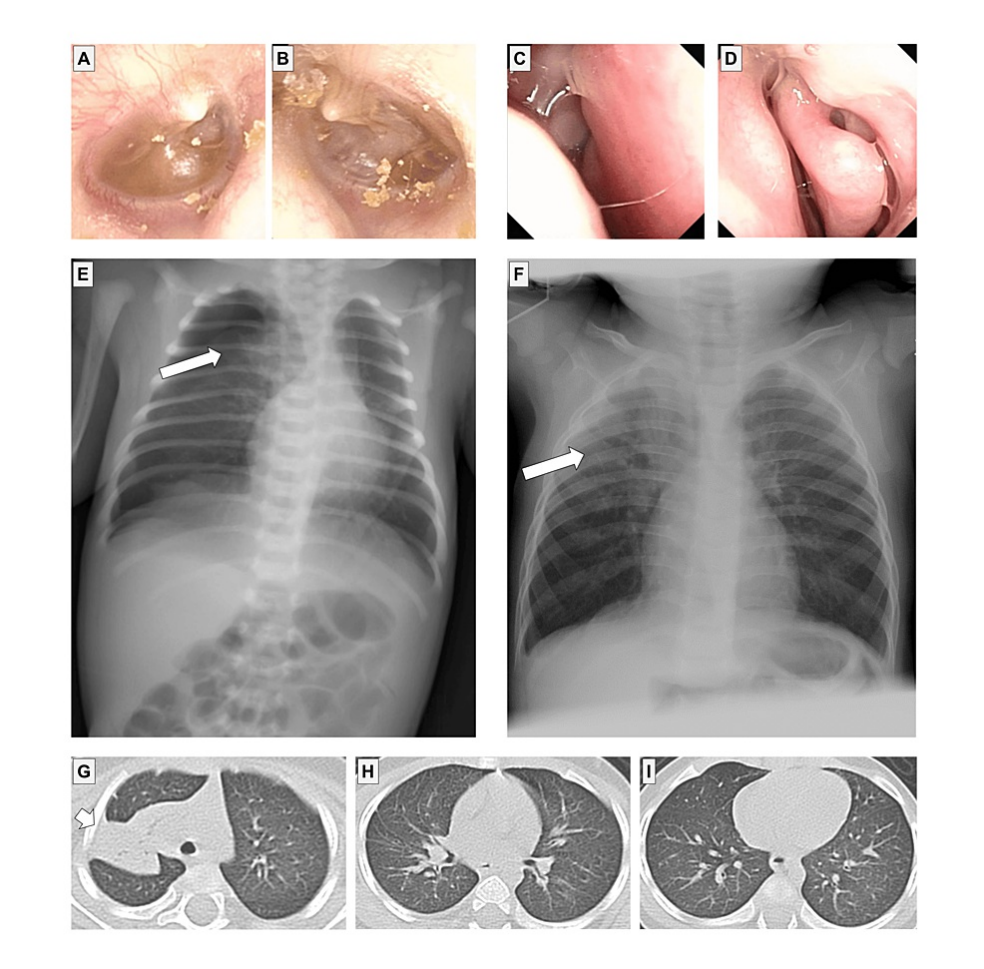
Imaging findings in Case 1. (A, B) Residual fluid and air-fluid levels in the bilateral middle ears. (C, D) Mucus secretion in the bilateral nasal cavities. (E) Chest X-rays immediately after birth and (F) at 10 months showed a shadow (arrows) in the right upper lobe. There was no situs inversus. (G) Chest computed tomography of the upper, (H) middle, and (I) lower lobes showed consolidation (arrow) in the right upper lobe.
